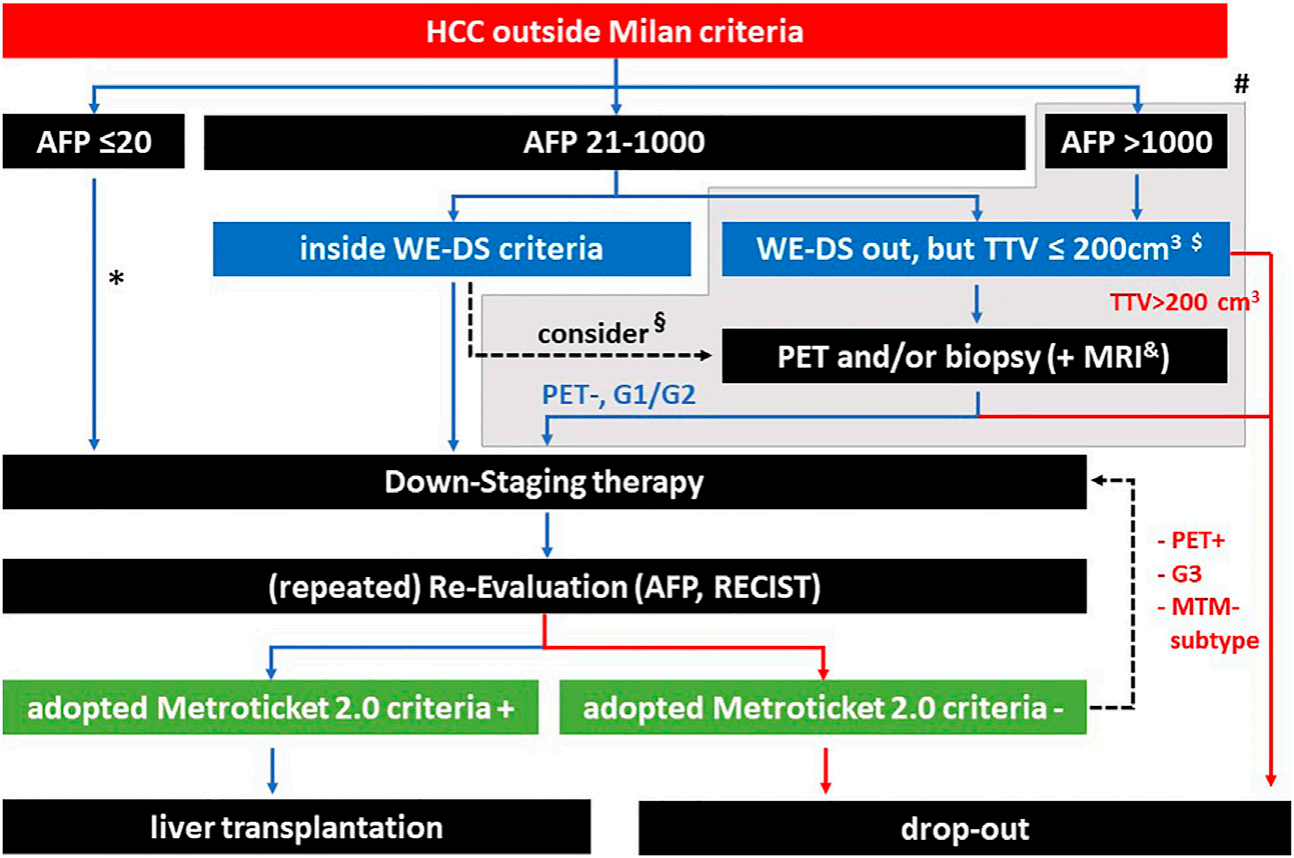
Summary of potential selection criteria before and during downstaging. * according to the WE-DS criteria, the upper limit of sum and number would be 12, above this value a PET/biopsy might be considered. § depending on the tumor burden, also inside the WE-DS criteria, additional information concerning tumor biology might be useful. # patients outside the WE-DS criteria (grey background) have a significantly elevated risk of drop out during waiting time, however, this does not preclude selection of a low percentage of patients with good prognosis after LT (depending on individual center policies and organ availability). $ with a TTV <200 cm^3^, the chance of successful downstaging is below 5% (Murali et al. [12]). & MRI techniques are evolving for the prediction of mVI and the macrotrabecular-massive (MTM) HCC subtype. Hepatobiliary MRI has proven higher sensitivity with comparable specificity for HCC depiction in cirrhotic patients.
